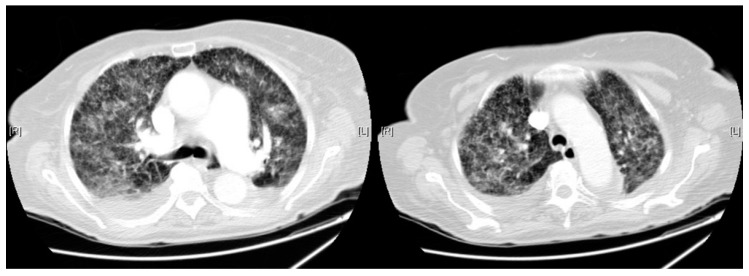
Chest CT on 10th day: Extensive pulmonary parenchymal involvement consisting of irregular septal thickenings with ground-glass areas and centrilobular nodules with a peri-lymphatic distribution.
